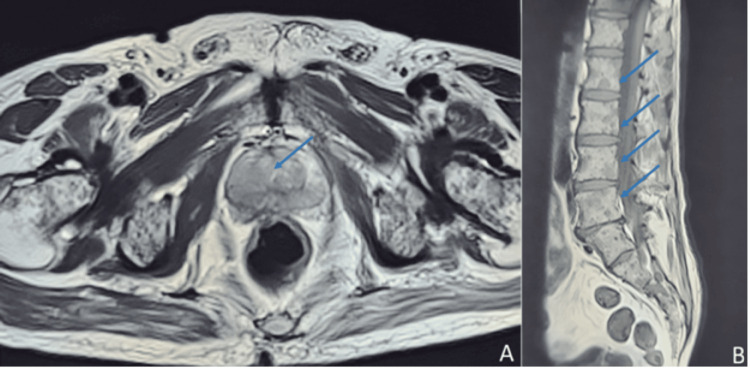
(A) Axial MRI image reveals enlarged prostate gland with evidence of irregular soft tissue lesion involving the peripheral zone on the right side, extending medially crossing the midline with involvement of left side of the prostate (blue arrow); (B) sagittal section MRI of lumbosacral spine reveals multifocal patchy to diffuse areas (blue arrows) of altered signal intensity lesions involving multiple lumbar vertebrae, suggestive of multiple lytic lesions.
